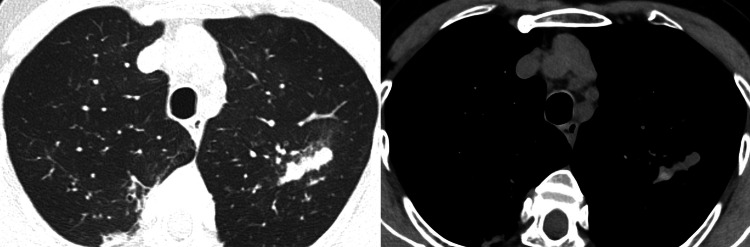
Computed tomography image of the chest when the patient was diagnosed with allergic bronchopulmonary aspergillosis
